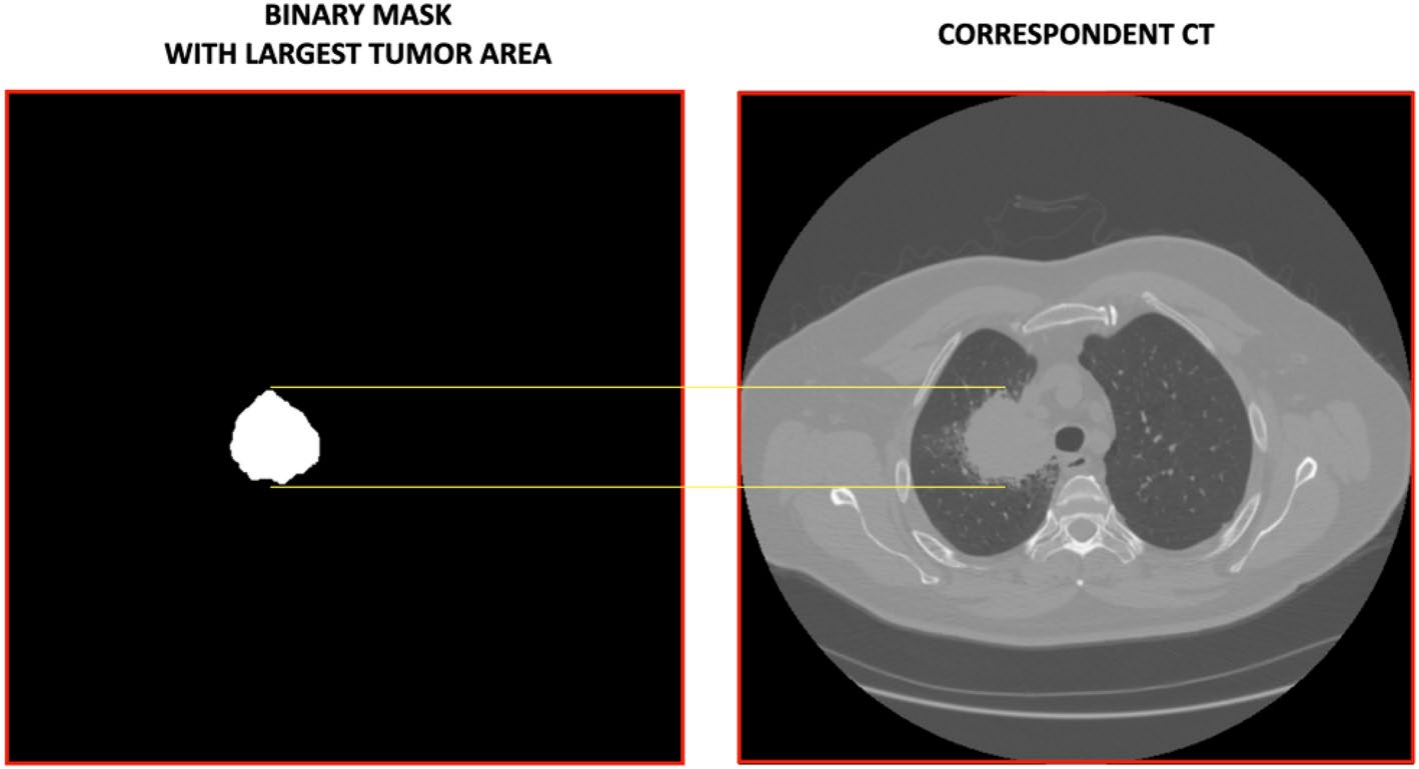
An example of a binary Mask with the largest tumor area and its corresponding CT are shown. The yellow lines mark the tumor area in the CT. For each patient, we detected this correspondence, and the CTs, suitably rescaled in the range [0;1] and with a specific input size, were then used as input for the ViTs, PVTs, Swins, and CNNs.
